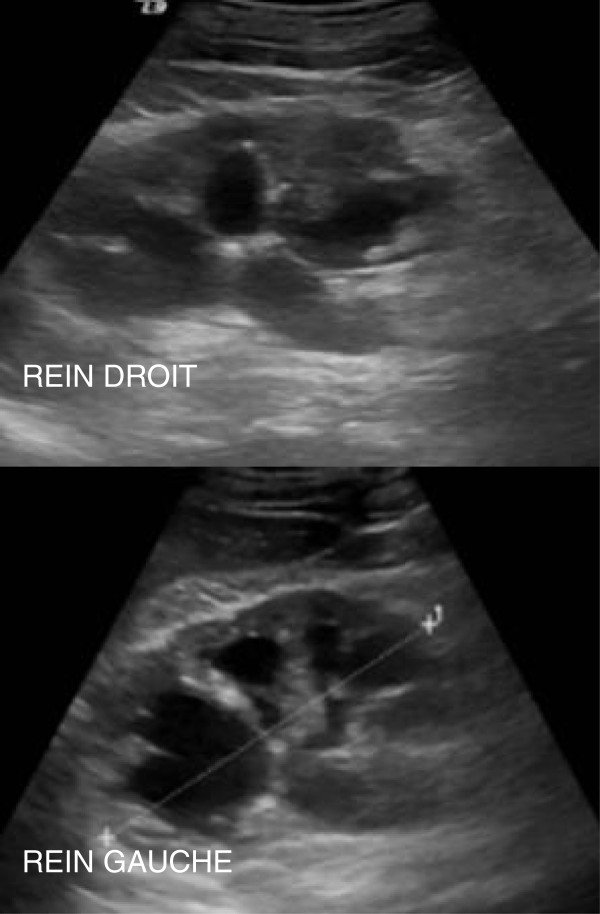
Renal ultrasound images showing two bilateral ureterohydronephroses. REIN DROIT: right kidney; REIN GAUCHE: left kidney.
